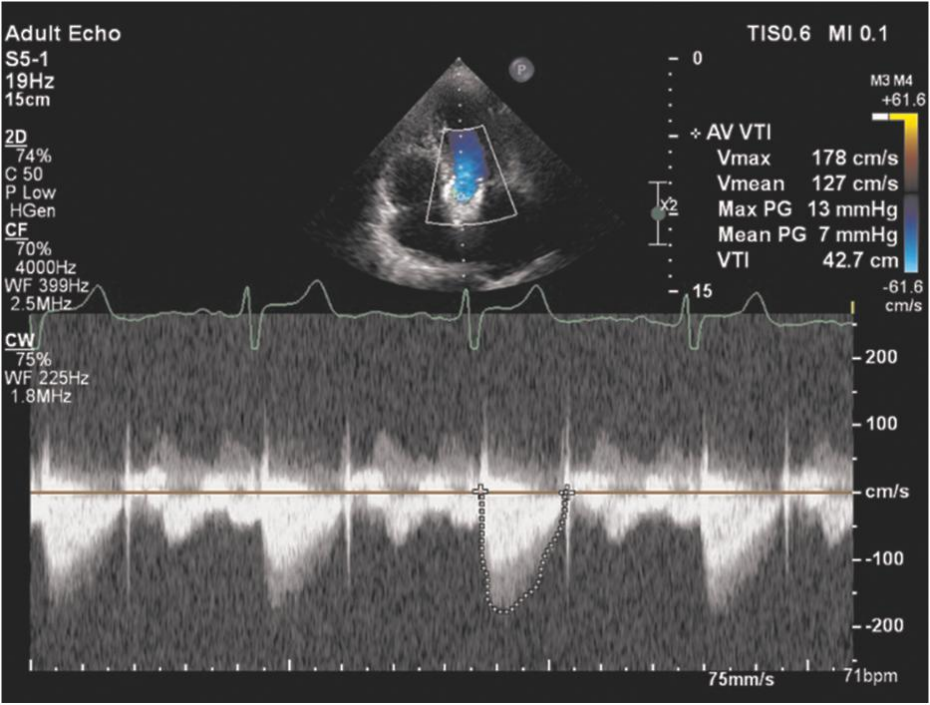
Follow-up transthoracic echocardiography after valve-in-valve transcatheter aortic valve implantation. Continuous-wave Doppler across the aortic valve demonstrates low peak and mean transvalvular gradients with no evidence of paravalvular regurgitation.
